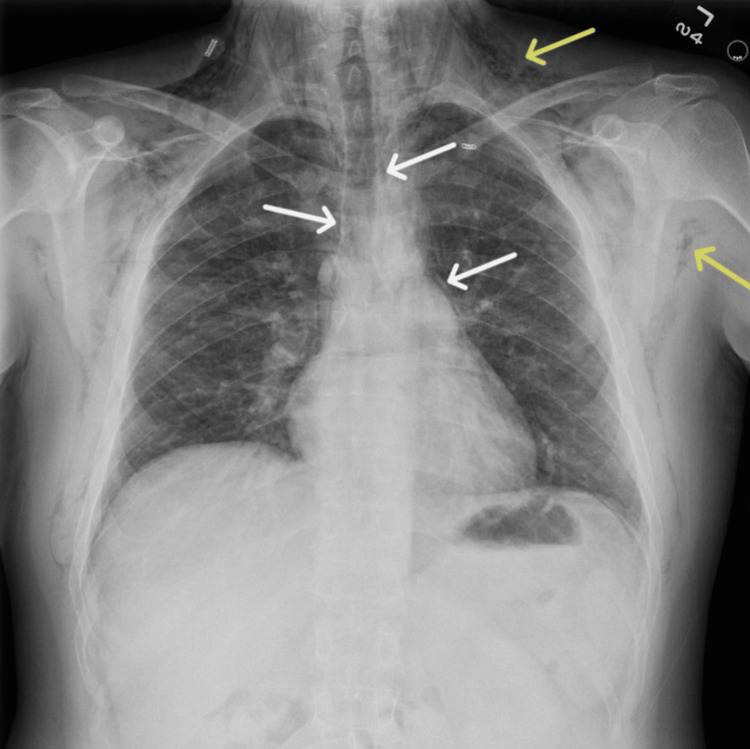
Posterior-anterior chest X-ray demonstrating pneumomediastinum (white arrows) and subcutaneous emphysema (yellow arrows).
